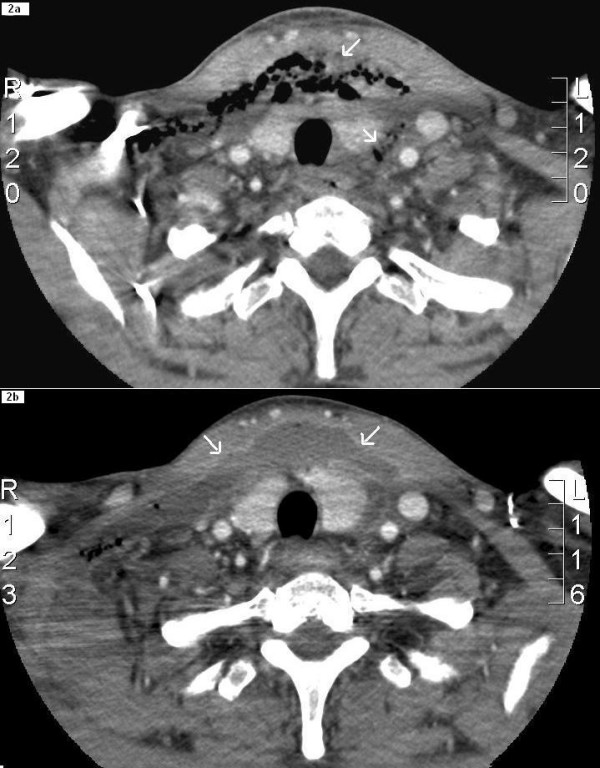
2a: Horizontal CT view of the neck at the level of suprasternal notch; the surgical emphysema and soft tissue swelling (arrows) is seen in the pretracheal region and prevertebral space and is extending beyond the carotid sheaths. In 2b (after 3 days of antibiotic therapy), the appearance of the disease process has changed (in comparison to 2a) with a decreasing amount of air in the soft tissues and replacement by an abscess (arrows) which has irregular enhancing margins.
